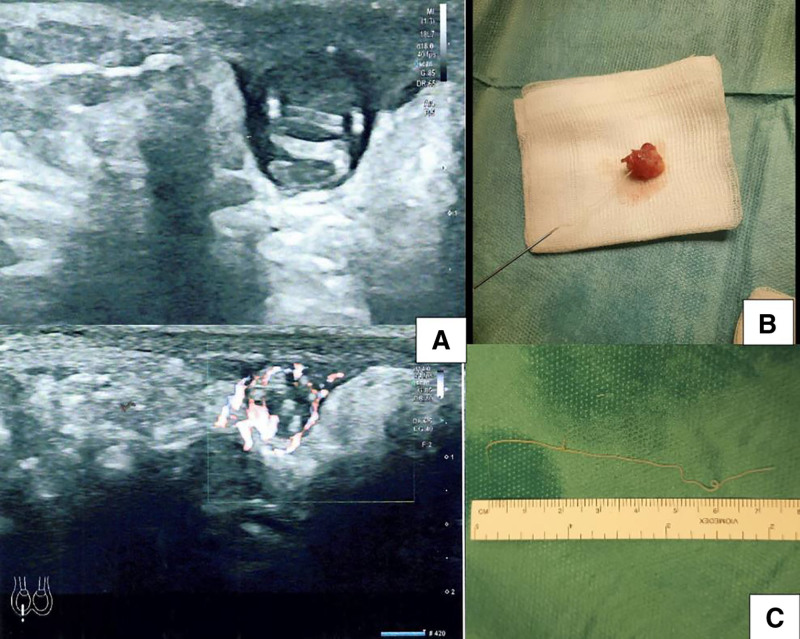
Ultrasonographic and macroscopic aspect of *Dirofilaria repens*. A: Scrotal ultrasound showing a 8 × 10 mm cyst with peripheral vascularity containing echogenic linear undulating structures. These structures have the peculiar movements characteristic of the filarial dance sign on real-time ultrasonography; (B) the nematode was extracted intact alive from the cystic nodule. It shows spontaneous movements; (C) mature female worm of Dirofilaria repens.
